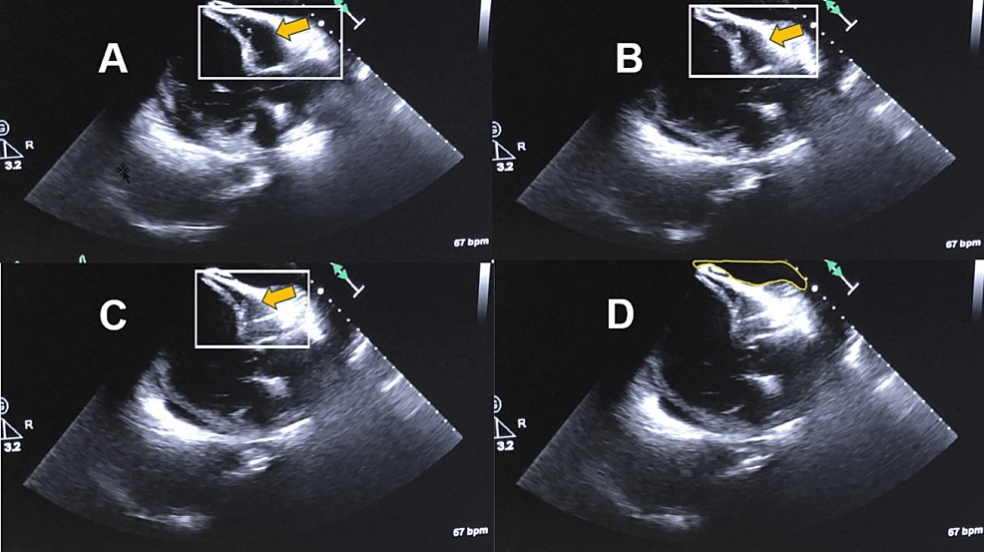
Echocardiogram (off-plane long axis view) with near complete right ventricle collapse (A, B, C) and surrounding pericardial effusion (D)
